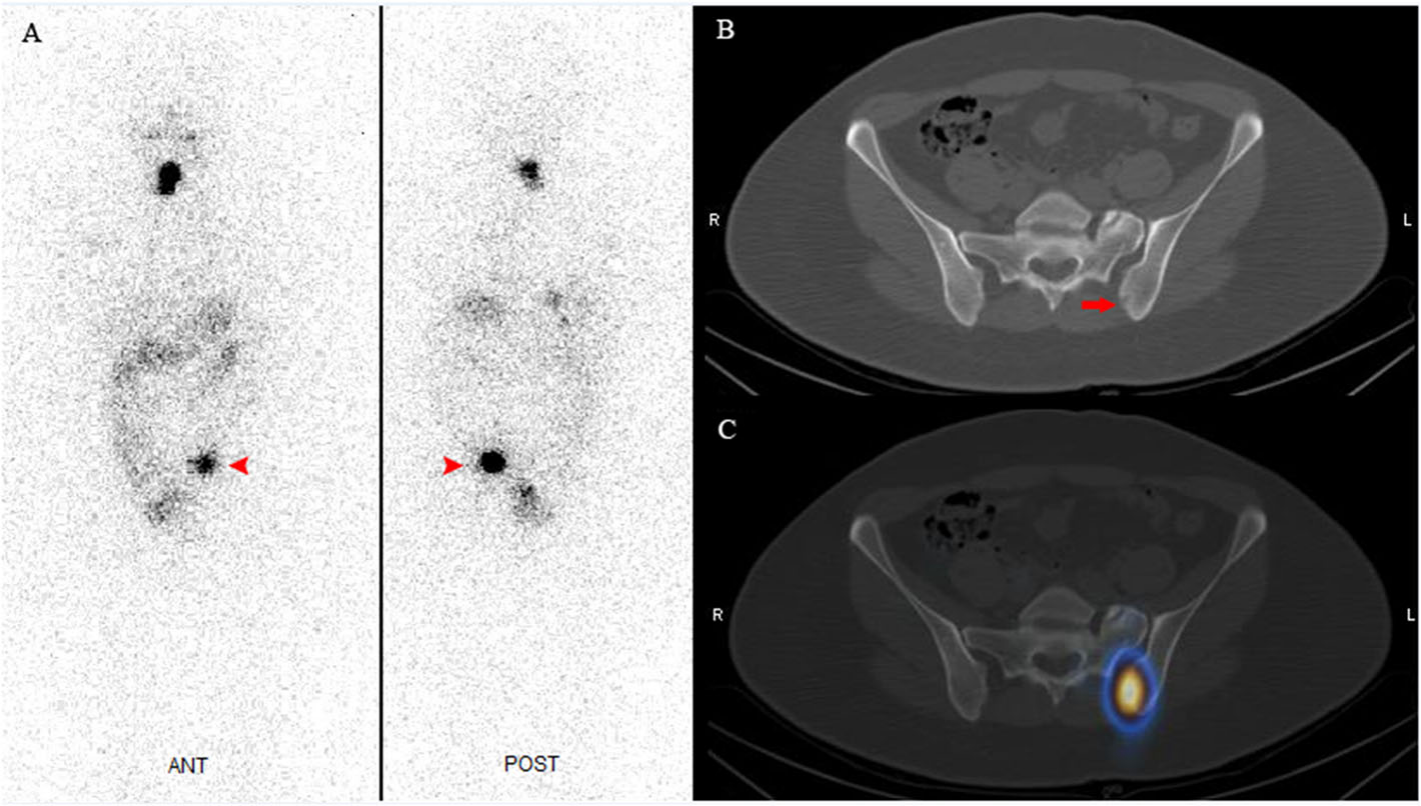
Diagnostic whole body iodine scan shows post-surgical thyroid remnant (PSTR) and a focus of abnormal uptake in the left hemipelvis (A; *arrowhead*). SPECT/CT from the pelvic region shows a lytic lesion in the left iliac bone (B; *arrow*), with intense iodine 131 uptake (C)
